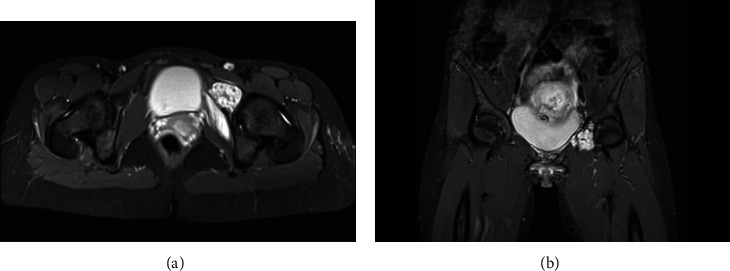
Axial (a) and coronal (b) T2 STIR weighted views on initial MRI of the pelvis showing a chondrogenic lesion of the left iliopubic ramus extending in the anterior acetabular wall.
